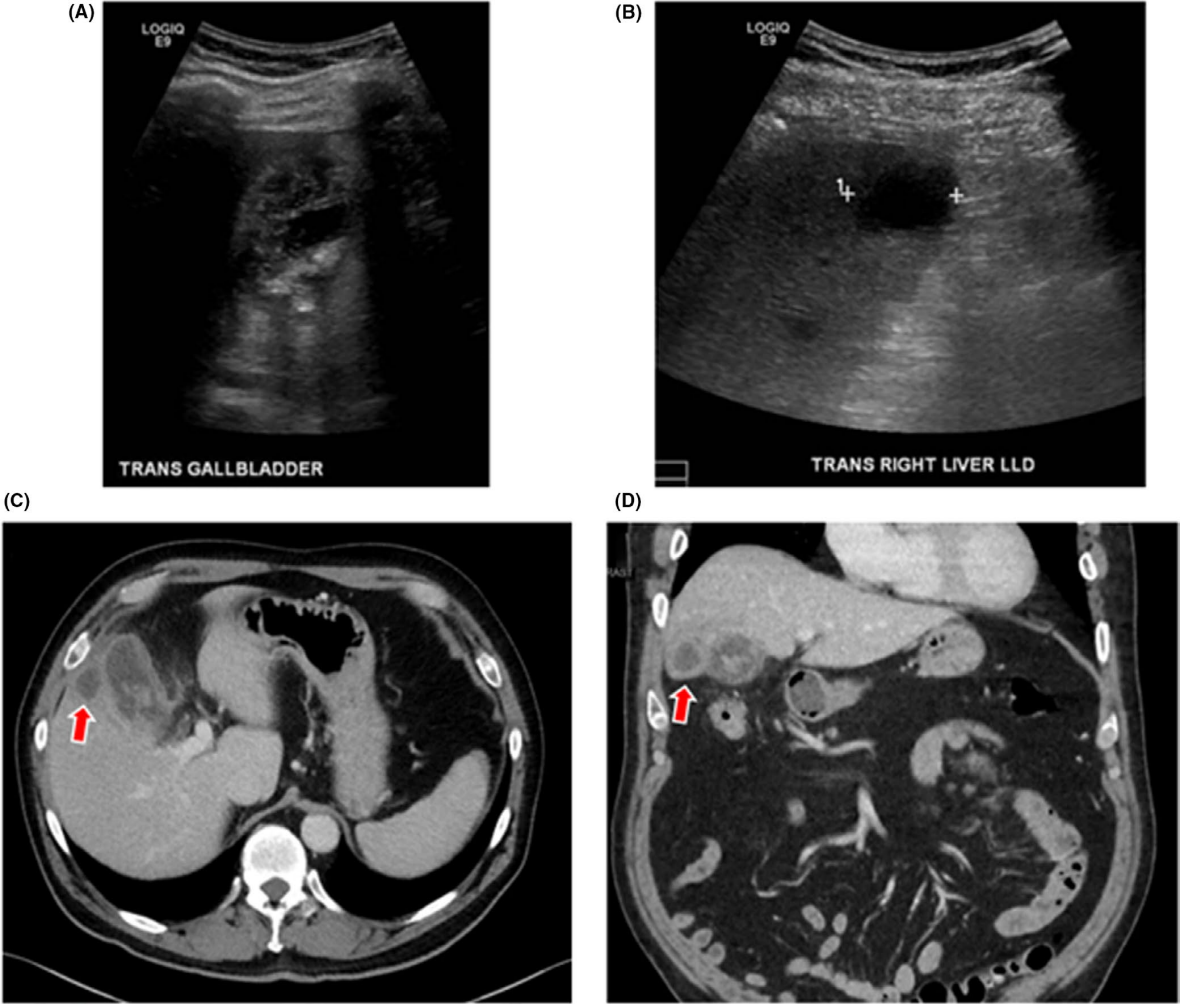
A 76‐year‐old man presented with minimal abdominal pain, nausea, emesis, and night sweats. Ultrasound showed cholelithiasis with thickened gall bladder (GB) walls (A) and 2.8 × 2.6 × 2 cm hypoechoic collection in the right hepatic lobe (B). Computed tomography scan showed thickened and irregular GB walls, pericholecystic stranding, and intraluminal membranes, suggestive of gangrenous cholecystitis (C,D). Fluid collection adjacent to GB (red arrows) is suggestive of pericholecystic abscess. The patient was successfully managed with percutaneous cholecystostomy
